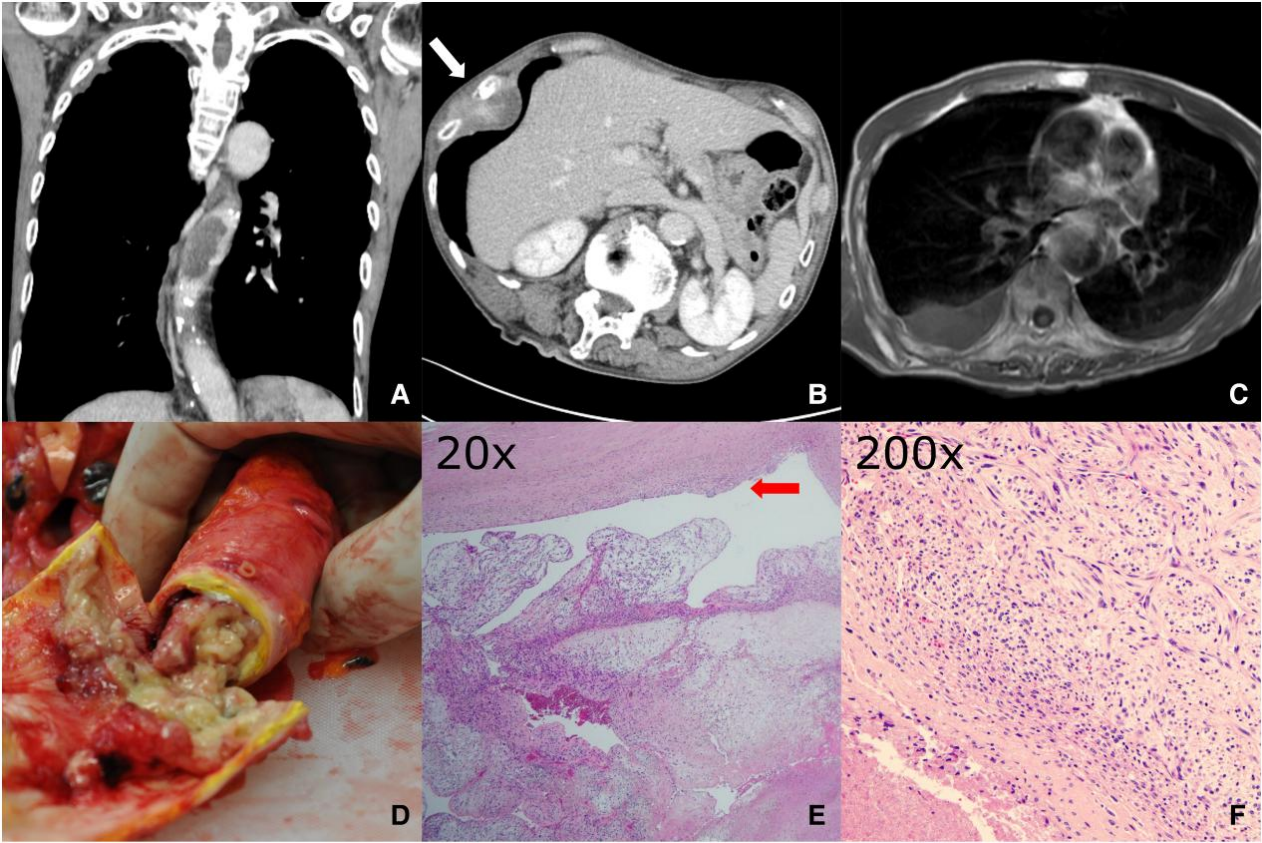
An 86-year-old man visited our hospital with right abdominal pain for 2 weeks. Contrast-enhanced computed tomography revealed a non-enhancing structure spanning > 55 mm in the thoracic descending aorta between the seventh and ninth thoracic vertebral levels (*Panel A*). Additionally, a heterogeneously enhanced mass (diameter, 65 mm) was observed on the right chest wall involving the eighth and ninth ribs (*Panel B*, white arrow). The intra-aortic structure was hyperintense on T1-weighted magnetic resonance images, indicating a thrombus in the descending aorta (*Panel C*). Needle biopsy of the chest wall lesion suggested spindle-cell carcinoma. The patient succumbed to disease progression 1 month after the initial visit, and an autopsy was performed. The right chest wall tumour was diagnosed as undifferentiated pleomorphic sarcoma (UPS), previously known as malignant fibrous histiocytoma. Atherosclerosis affected the aorta, extending from the thorax to the abdomen. In the descending thoracic aorta, a yellowish-white soft lesion occupied the lumen for >90 mm (*Panel D*), consisting of tumour emboli of proliferating UPS cells and necrosis (*Panels E*; red arrow, the aortic wall; *Panel F*). The tumour lesions extended from the vascular intima that was affected by the atheroma, and the elastic fibres in the tunica media were ruptured or missing. Although intimal metastases in the aorta have rarely been reported, possibly due to the rapid blood flow, factors affecting intimal conditions, such as arteriosclerosis, may promote intimal attachment of malignant cells derived from other organs.
